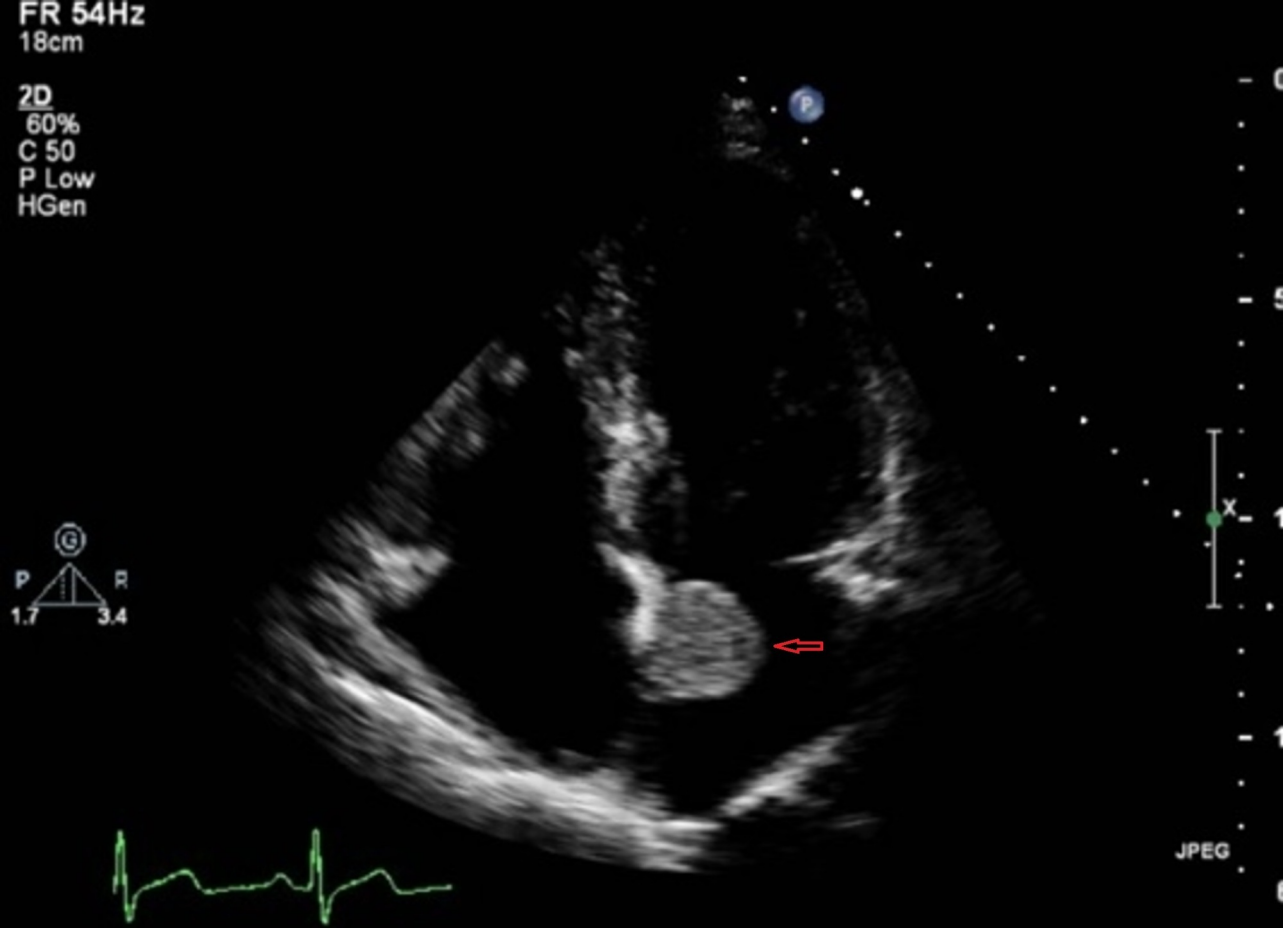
TTE frame shows left atrial myxoma. Red arrow points to left atrial myxoma. TTE: Trans-thoracic echocardiogram.
